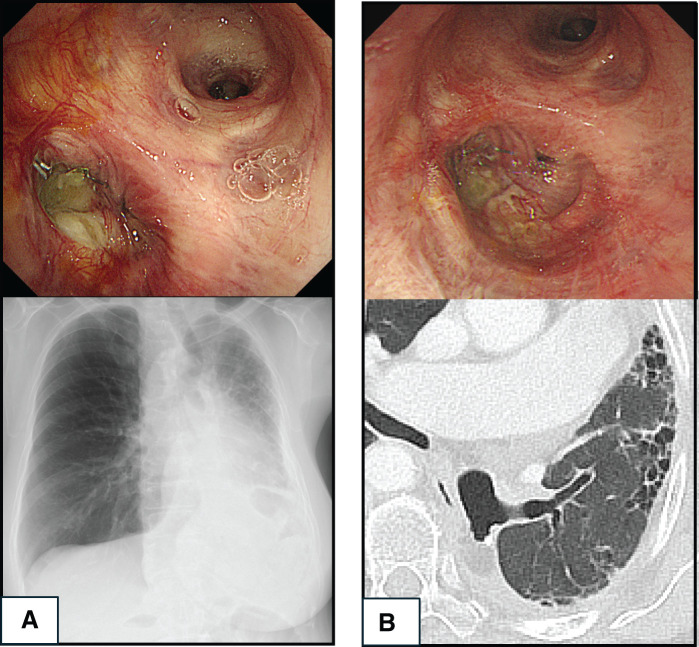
(A) Bronchoscopy on POD 90, revealing staple line dehiscence and fat pad exposure, consistent with BPF. Chest radiography showed no pneumothorax or air-fluid levels. (B) Bronchoscopy on POD 153, demonstrating epithelialization and fistula improvement. CT confirmed upper lobe re-expansion with no peristump air. Red dotted line indicates the fat pad overlying the bronchial stump. BPF, bronchopleural fistula
